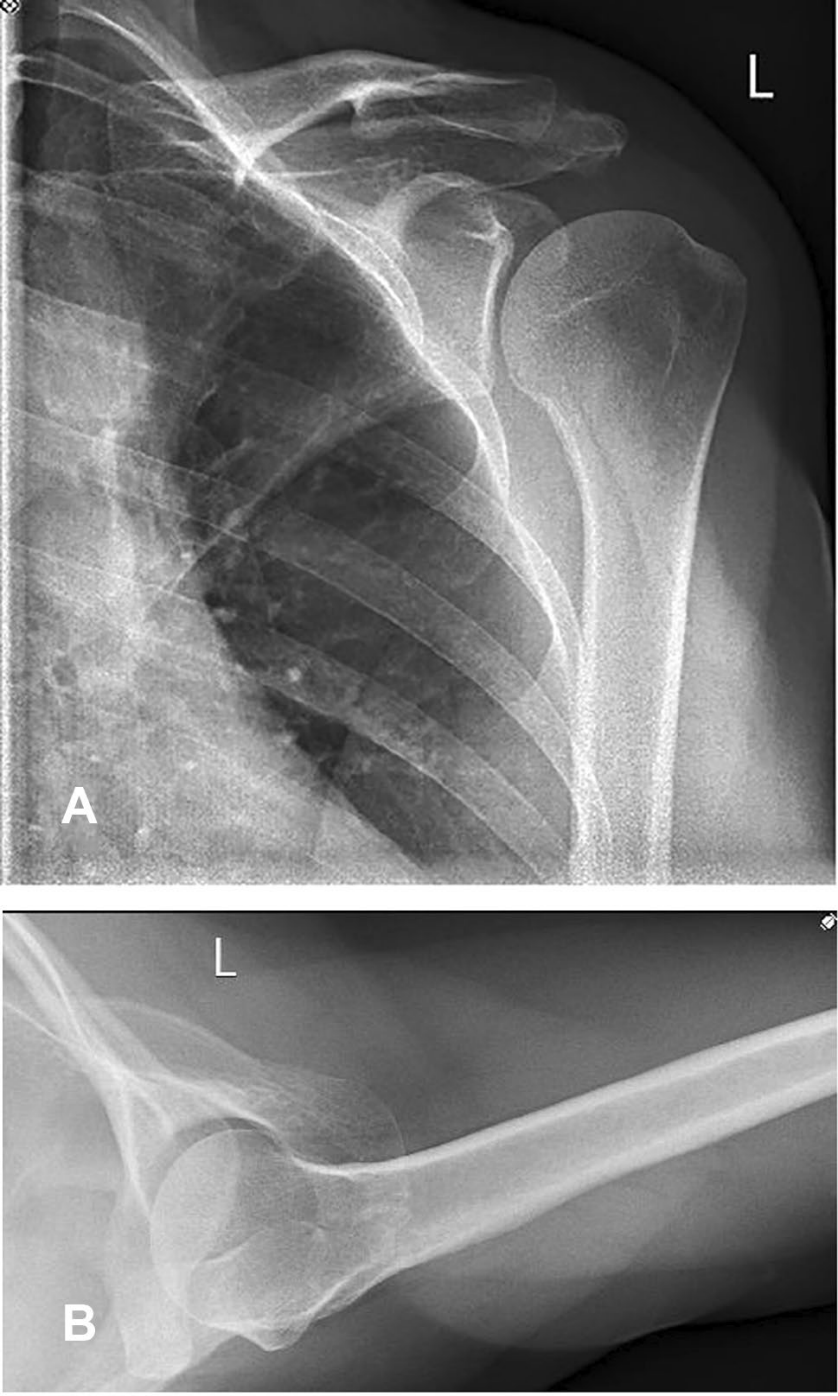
A–B Preoperative anteroposterior and axillary view radiographs
